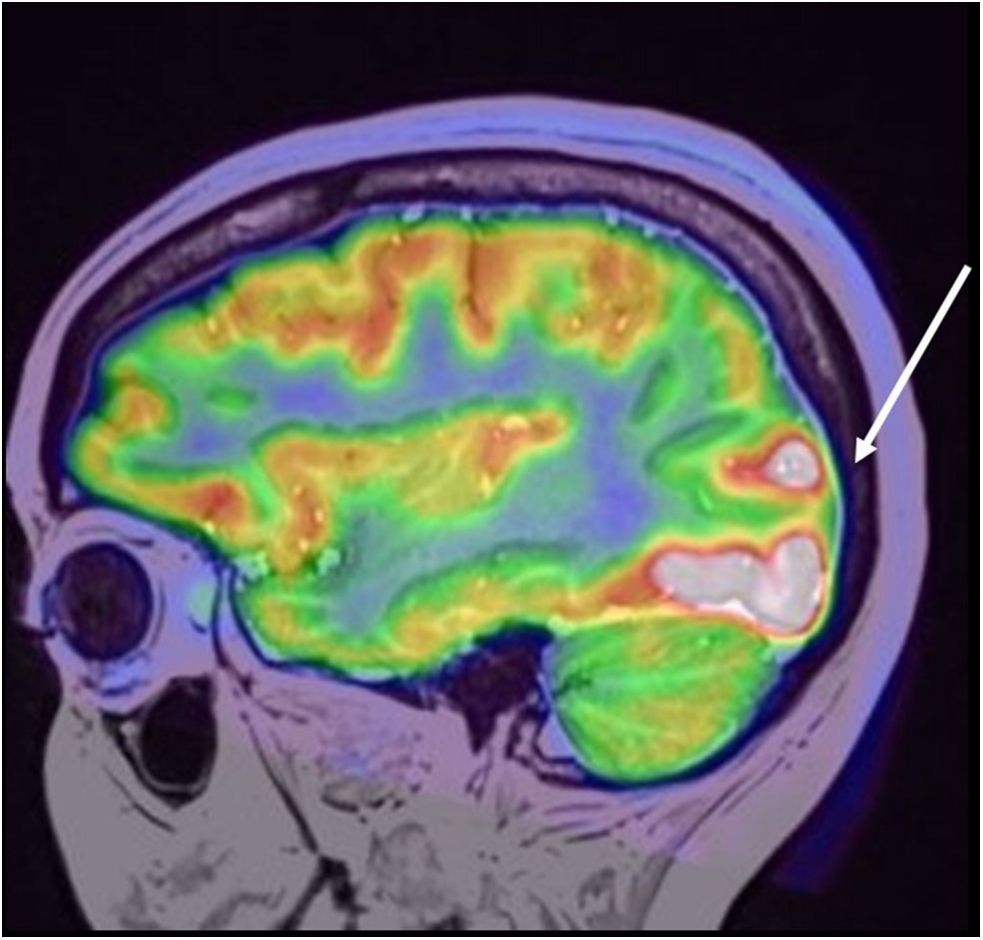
Coregistered PET/MRI study, depicting focally increased metabolic uptake in the right occipital lobe involving the lateral gyri with extension to cuneus and lingual gyri. No contralateral uptake was found or any other foci or abnormal metabolic uptake in the brain or whole body.
